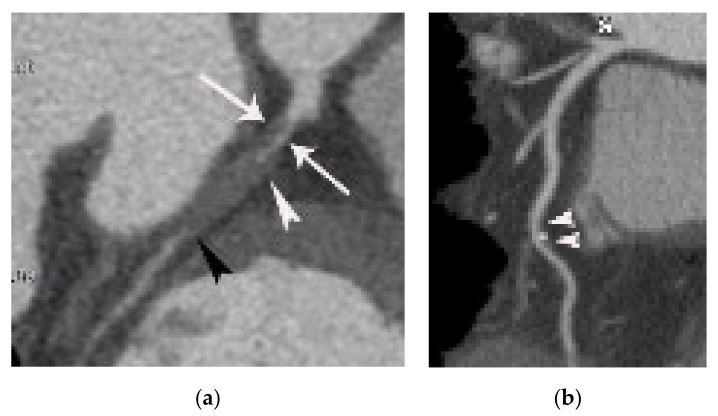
Difference between culprit (a) and nonculprit plaque (b) on coronary CTA. (a) Note the total occlusion (arrowheads) by noncalcified plaque with vulnerable CT features (positive remodeling and napkin ring sign appearance (arrows)) in the proximal left circumflex artery in a patient with AMI, showing it to be the culprit lesion. (b) In contrast, <50% stenosis with mixed plaque without vulnerable CT features (arrowheads) is noted in the mid left anterior descending coronary artery in a nonculprit lesion.
